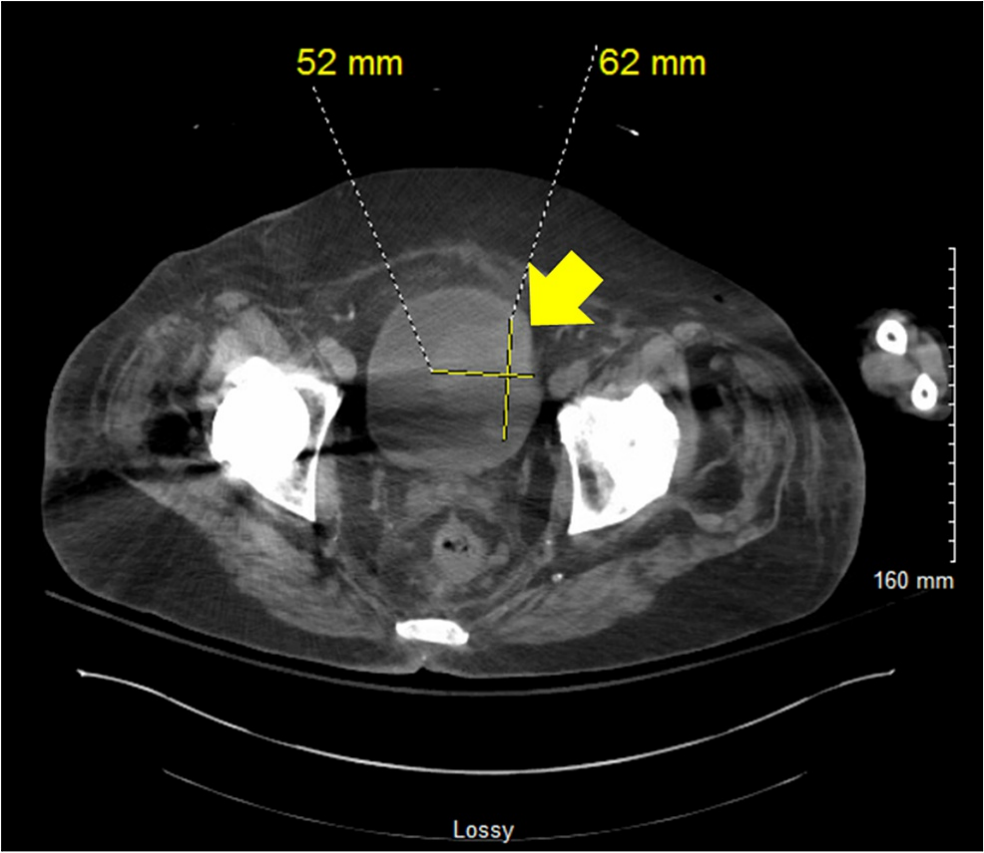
Computed tomography (CT) of the abdomen and pelvis without contrast showed a lobular mass along the left side wall of the urinary bladder measuring 5.2 cm (yellow arrow) with irregular thickening, raising concern for a neoplastic process
